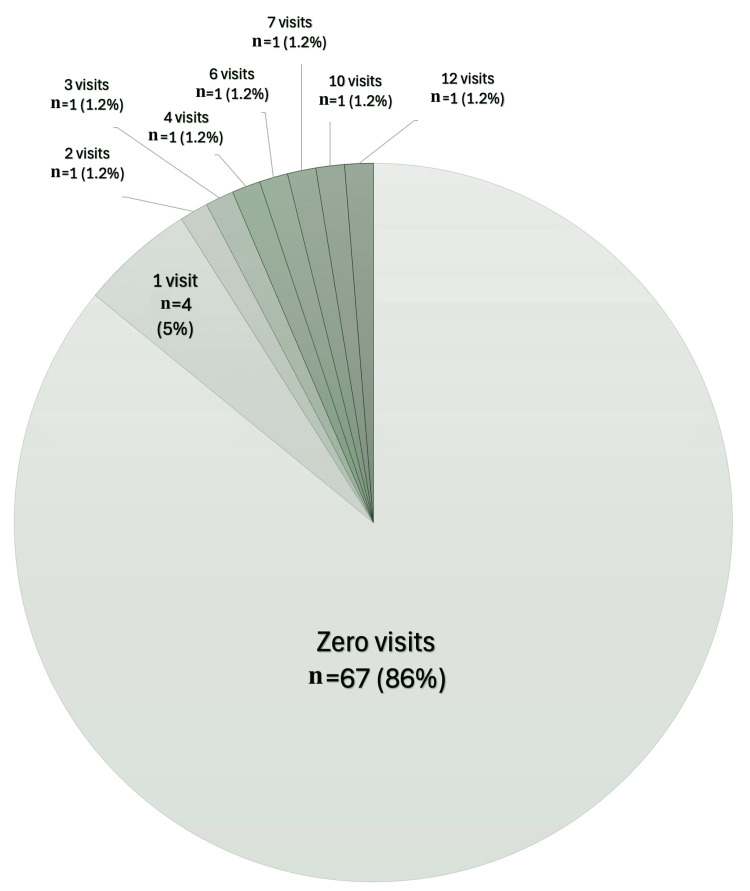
OT visits for uTFR-only patients. OT: occupational therapy; uTFR: ultrasound trigger finger release; n: number of patients
